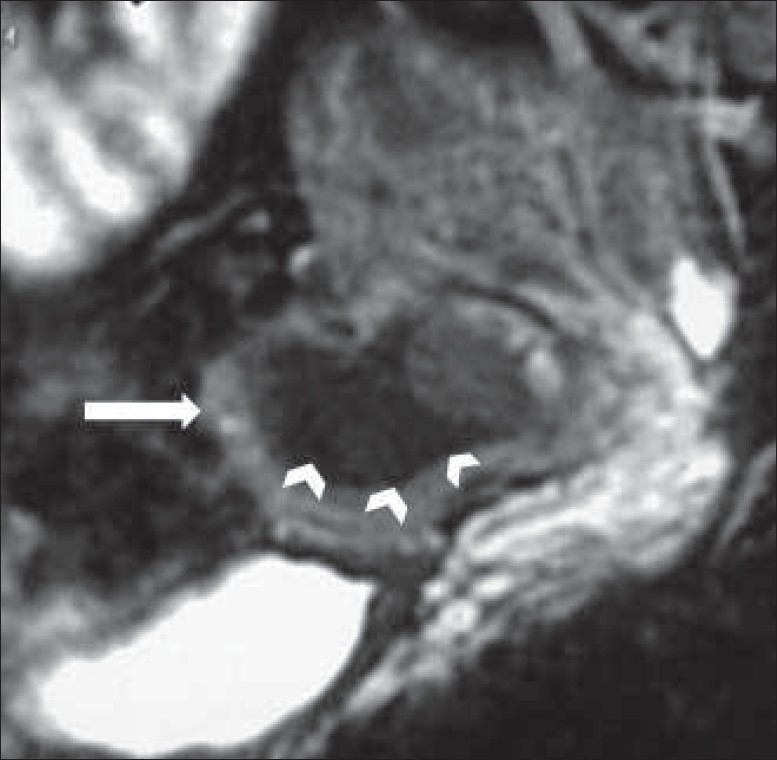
Sagittal T2W MRI image shows the anterior lower segment uterine mass (arrow) with the presence of blood products (arrowheads)
